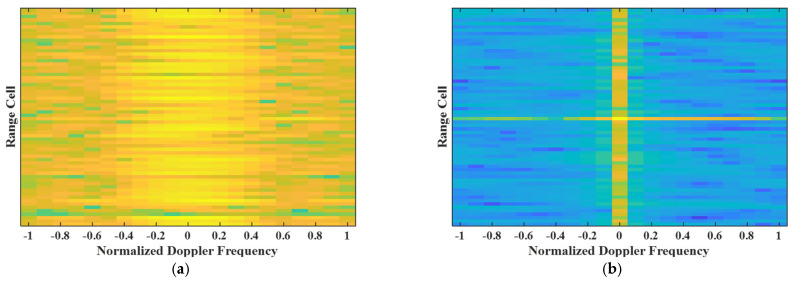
Range–Doppler spectra before and after clutter rejection: (a) before clutter rejection; (b) after clutter rejection.
